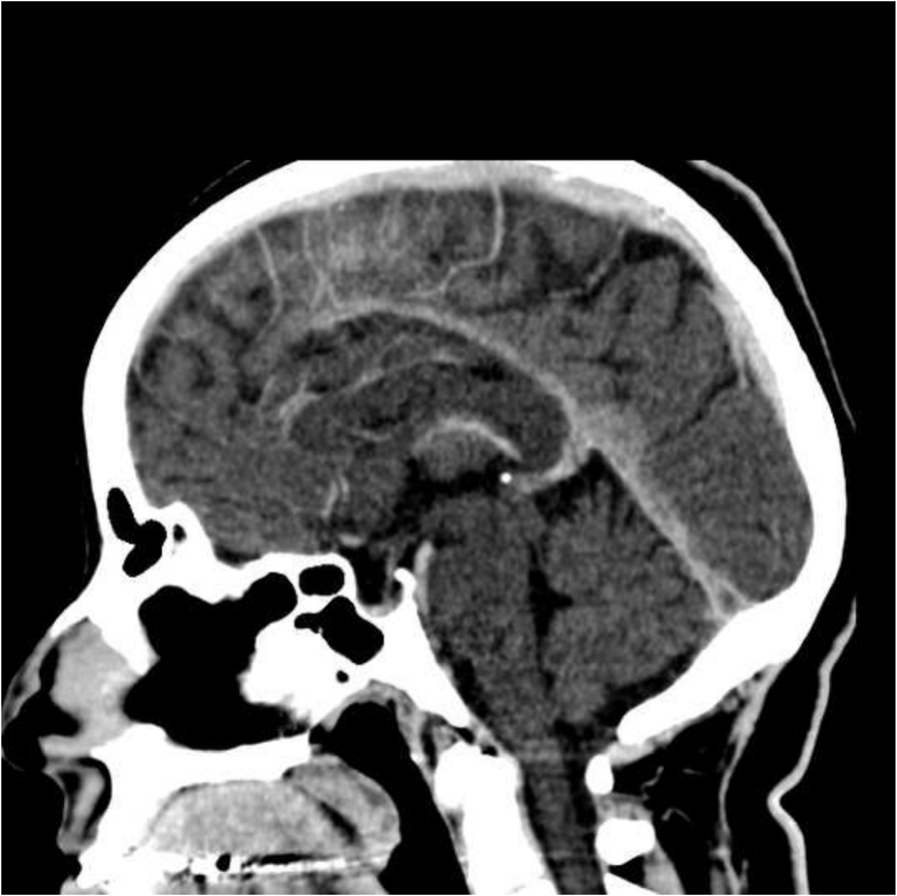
A sagittal view of the computed tomography brain with contrast showing the filling defect of the superior sagittal sinus that reassembles a triangle shape, called empty triangle or empty delta sign
